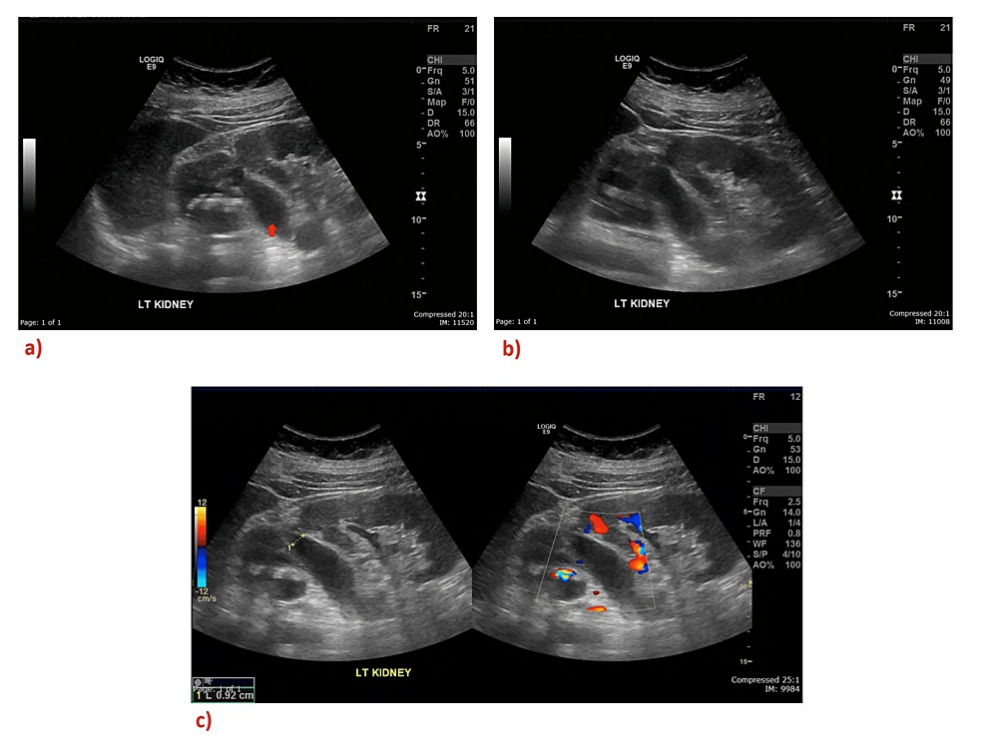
Selected images from the ultrasound abdomen and pelvis demonstrating a duplex renal collecting system with hydronephrosis and hydroureter on the left kidney Images A and B show a dilated left upper renal pelvicalyceal system (red arrow). Image C reveals the absence of color flow with a diameter of 0.92 cm, confirming a dilated pelvicalyceal system.
